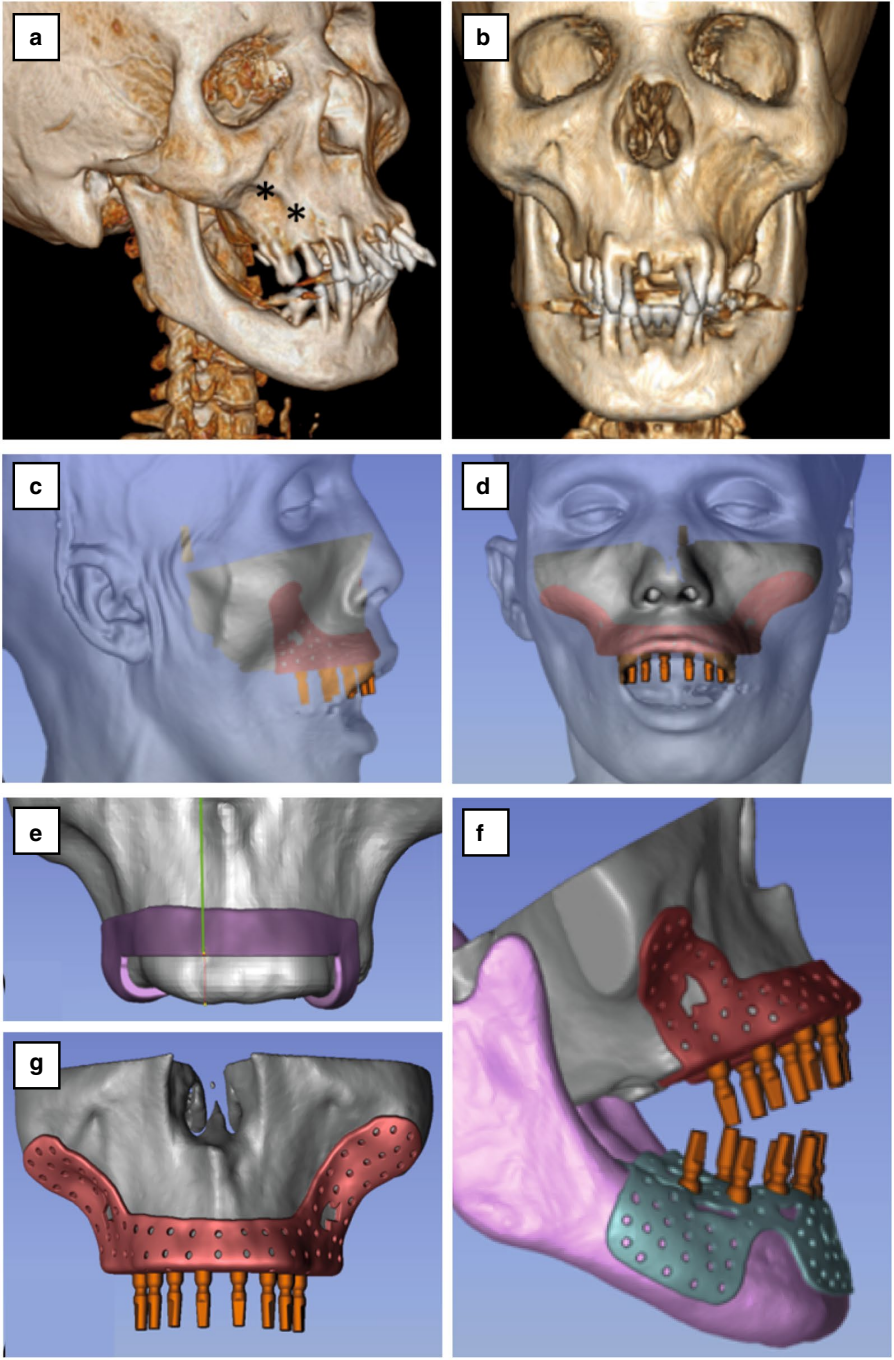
3D computed tomography scan of the patient’s skull. a,b Frontal lateral view showing prominent overgrowth of maxilla. *Periodontal (gum) disease. c Lateral 3D view and d frontal 3D view of soft tissue superimposed with hard tissue. Surgical plan. e Design level of osteotomy in maxilla. f Design of implants in maxilla and mandible with occlusion relation design of implant maxilla. g Design of implants in maxilla and mandible with occlusion relation and design of implant maxilla
